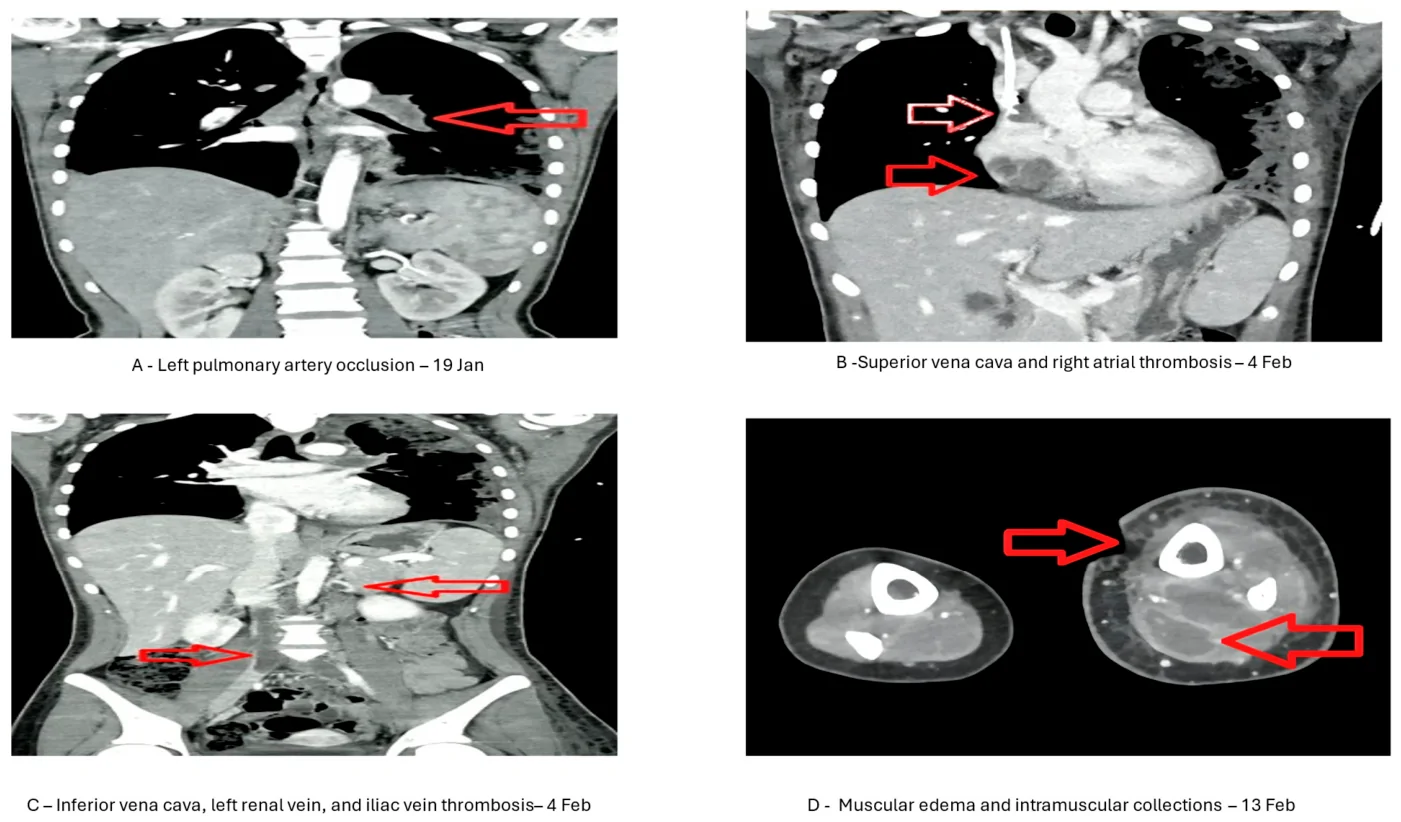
Serial contrast-enhanced CT images demonstrate progressive multisite thrombo-inflammatory disease despite therapeutic anticoagulation. (A) CT pulmonary angiography performed on 19 January 2026 showing complete occlusion of the left pulmonary artery with associated pulmonary infarction. (B) Coronal contrast-enhanced CT performed on 4 February 2026 showing thrombi involving the superior vena cava and right atrium. (C) Coronal contrast-enhanced CT performed on 4 February 2026 showing thrombosis of the inferior vena cava, left renal vein, and iliac vein. (D) Axial contrast-enhanced CT performed on 13 February 2026 showing diffuse muscular edema and multiple rim-enhancing intramuscular collections concerning for secondary infection. The arterial axis remained patent. Red arrows indicate the relevant radiological findings.
